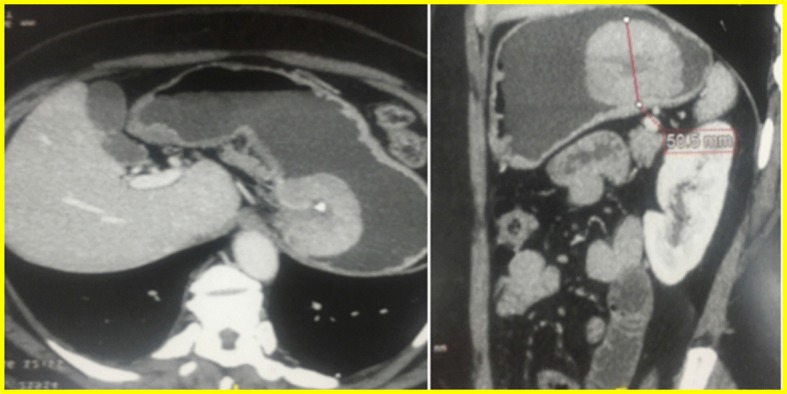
CT scan showing the tumour in the cardia
